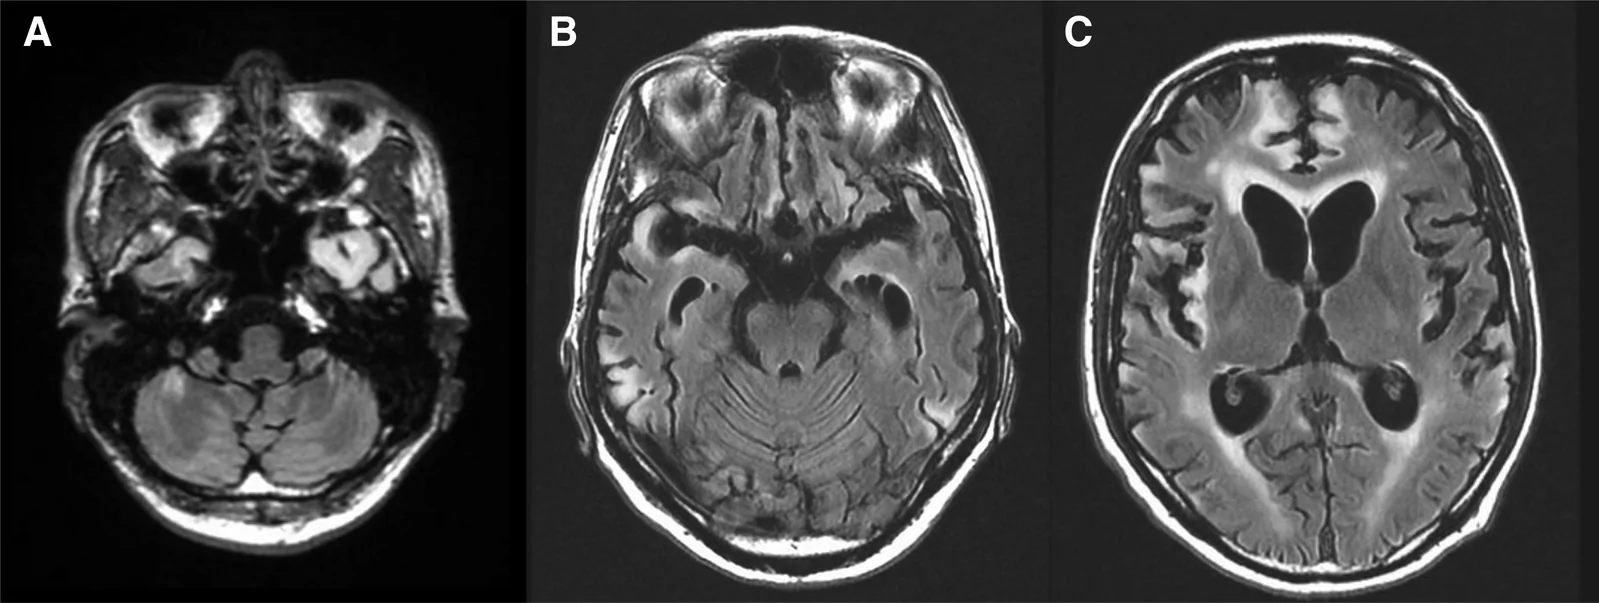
Brain magnetic resonance imaging on day 95 after onset. Axial fluid-attenuated inversion recovery images (A–C) demonstrate enlargement and increased conspicuity of cortical hyperintense lesions, with additional involvement of the insular cortex, cerebellum, and periventricular regions. Cerebral cortical atrophy and ventricular enlargement are also present.
